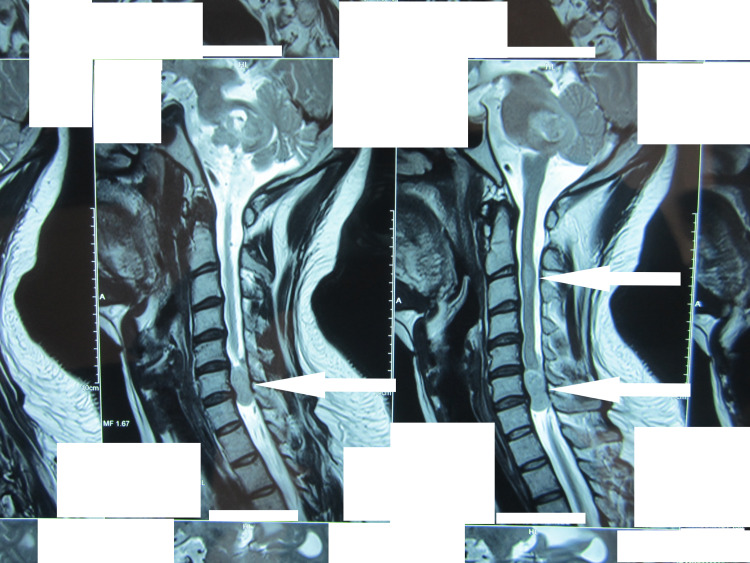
MRI showing multiple tumors in the cervical region of the spinal cord (arrows) MRI: magnetic resonance imaging
